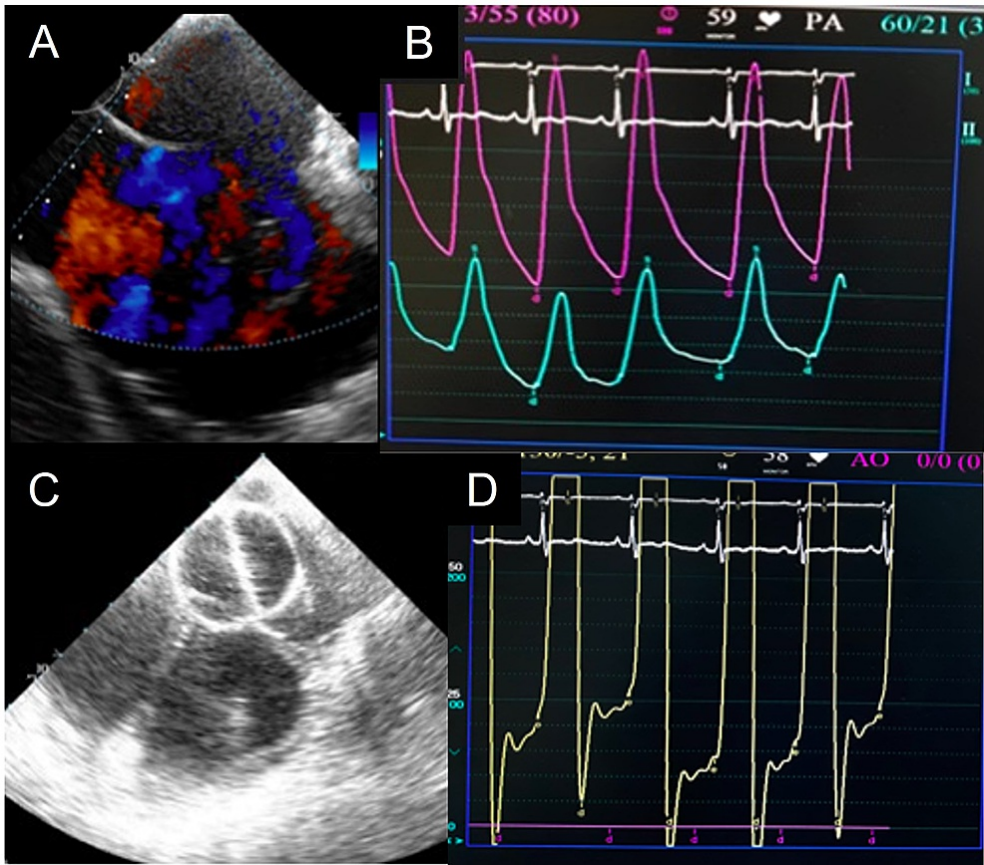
(a) Transesophageal echocardiography (TEE) with color Doppler showing large atrial septal defect with predominantly left-to-right (bidirectional shunt). (b) Basal aortic and pulmonary artery pressure. (c) TEE showing Equalizer balloon occlusion of the defect. (d) Post balloon occlusion elevated left ventricular end-diastolic pressure (50 scales).
